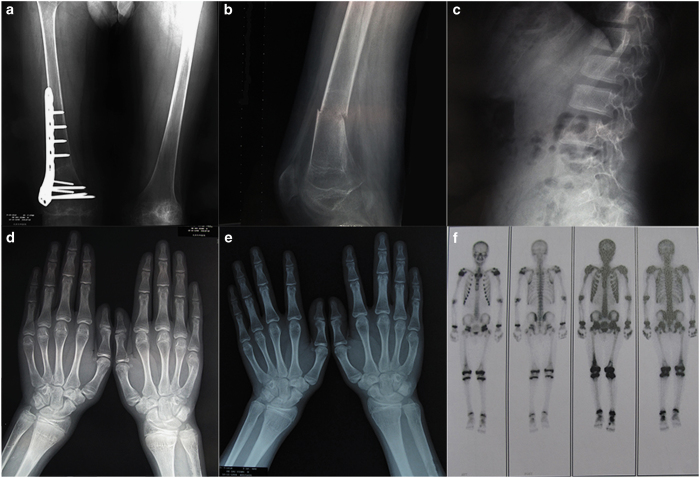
Radiographs and bone scans of a Chinese patient with familial hypocalciuric hypercalcemia. Radiographs of (a) orthophoria of the femur (postoperation), (b) lateral film of the femur (nonunion of fracture), and (c) lateral film of the lumbar vertebra showing osteoporosis. X-ray of the hands indicating epiphyseal broadening before (d) and after (e) epiphyseal closure. (f) Bone scan showing no specific foci of abnormal tracer deposition.
